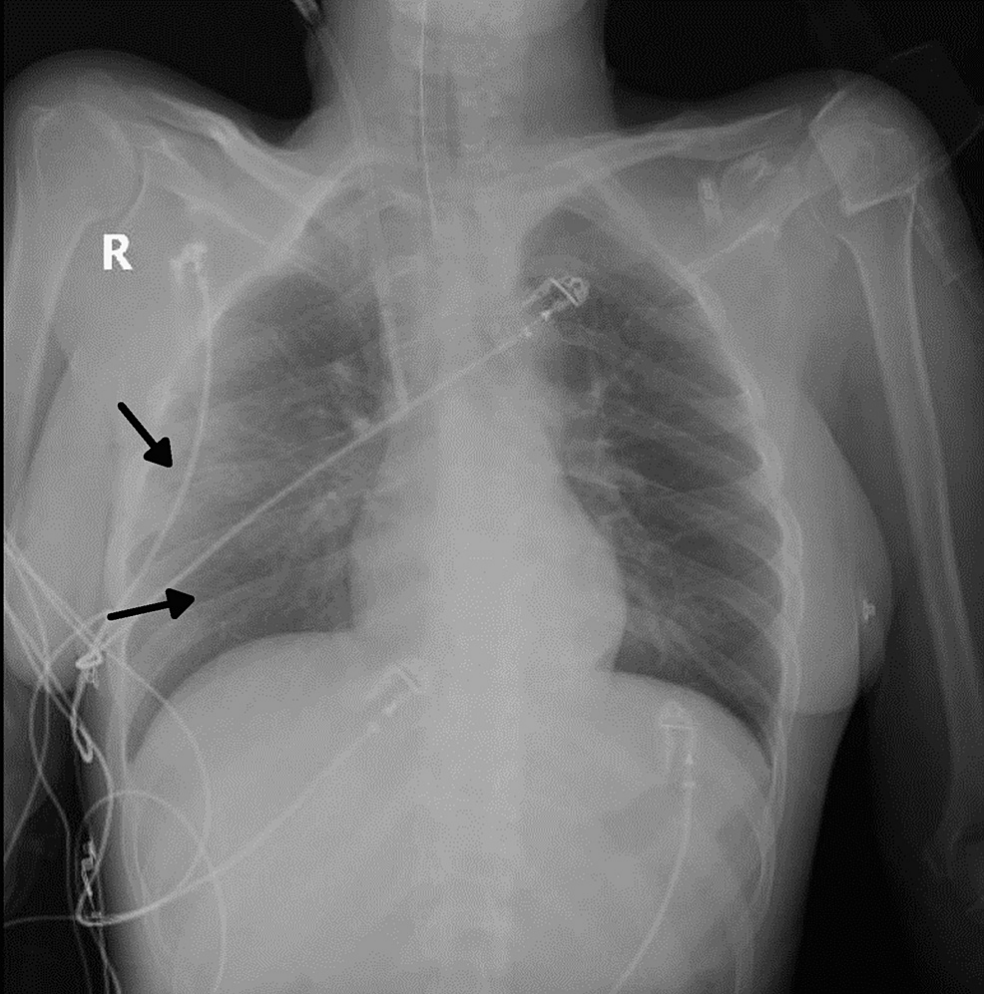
Chest X-ray posteroanterior view showing ground-glass opacities (arrows) in both the middle and lower lobes of the right lung.
